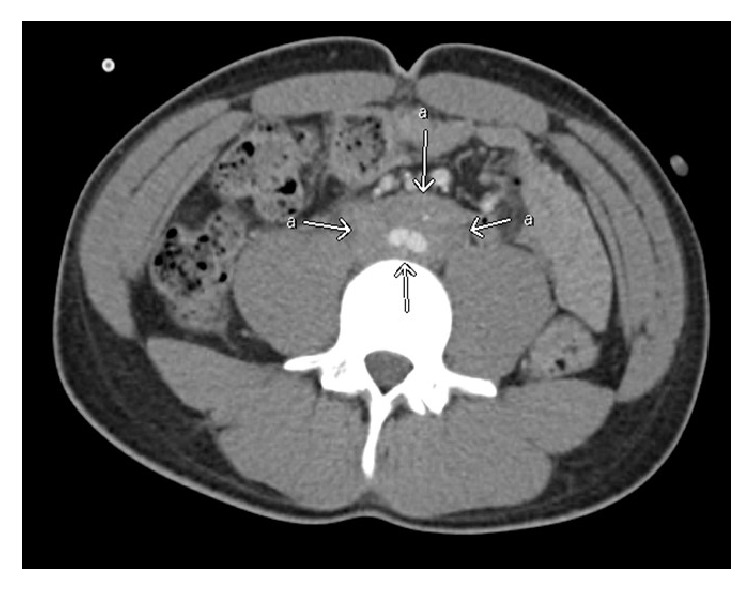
Abdomen and pelvis computed tomogram with intravenous contrast revealed mild bilateral hydroureteronephrosis with point of obstruction at the level of distal abdominal aorta and inferior vena cava. There was an ill-defined, infiltrative soft tissue mass encasing both aorta and IVC (approximately 7.2 cm). The mass appeared to infiltrate along the bilateral proximal common iliac vessels.
